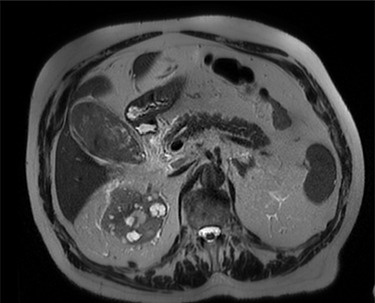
MRI scan of the patient, showing a distended gallbladder with low T1 and T2 content demonstrating no internal enhancement.
